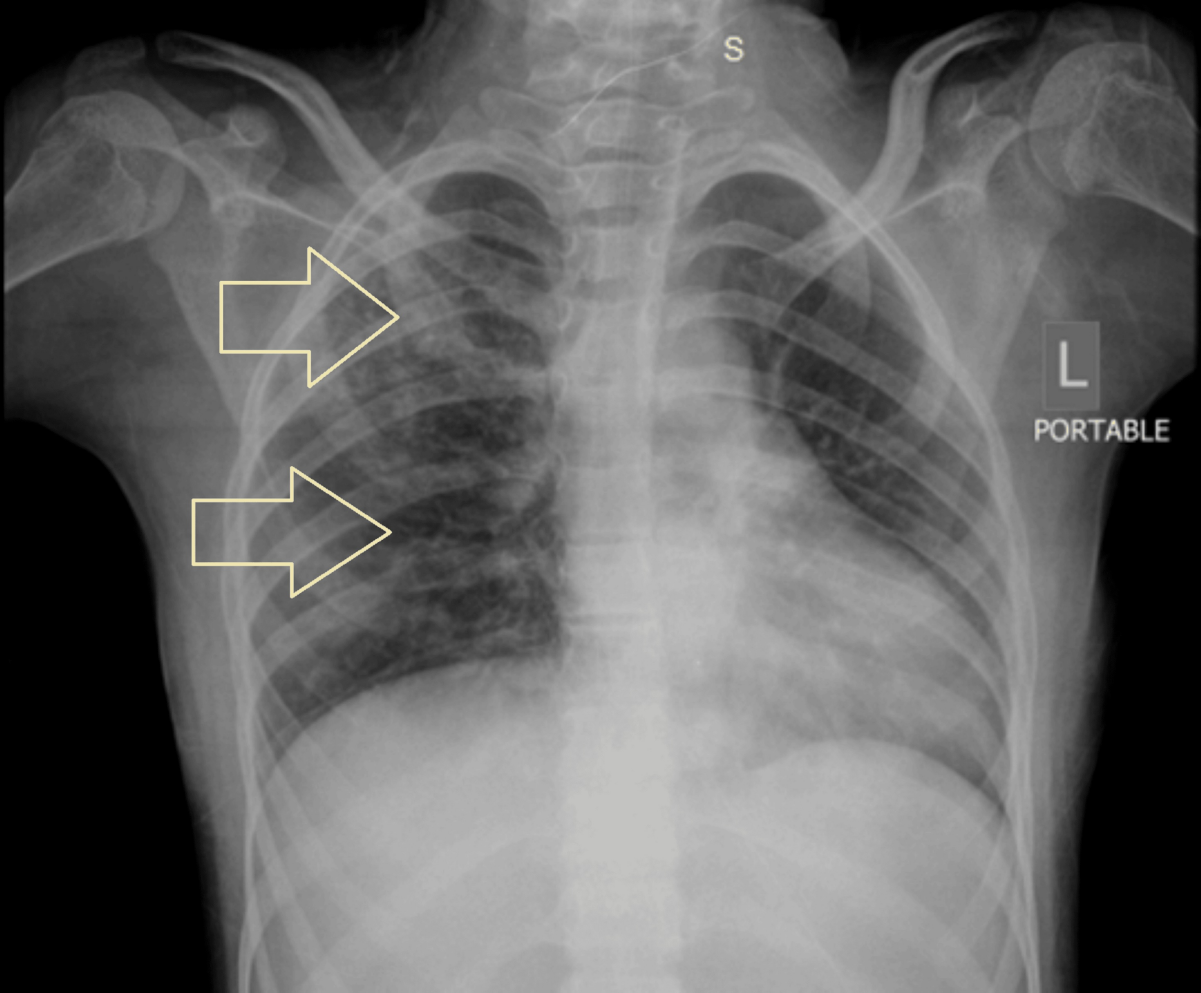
Chest X-ray at the time of admission showing ground-glass haze with infiltrates identified in the right upper and mid zones (white arrow)
